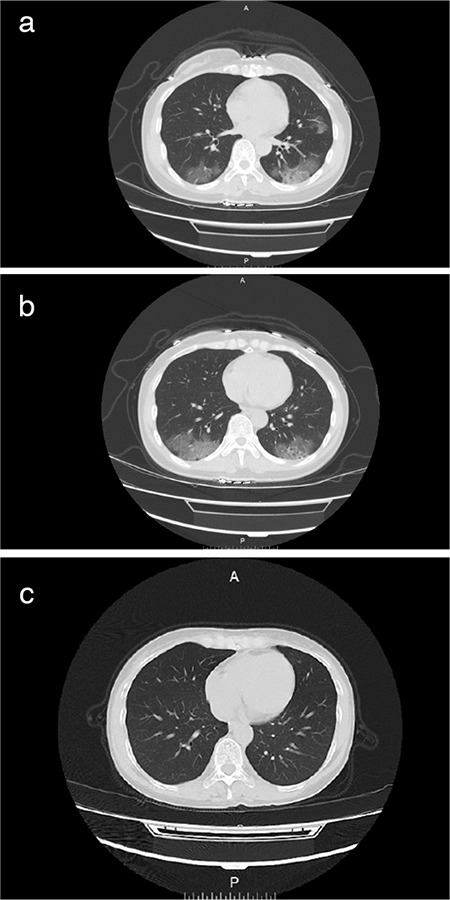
a., b. CT images reveal multifocal ground glass opacities with consolidation related with 2019 novel coronavirus (2019-nCoV). c. The infection foci of both lungs were absorbed and disappeared.
